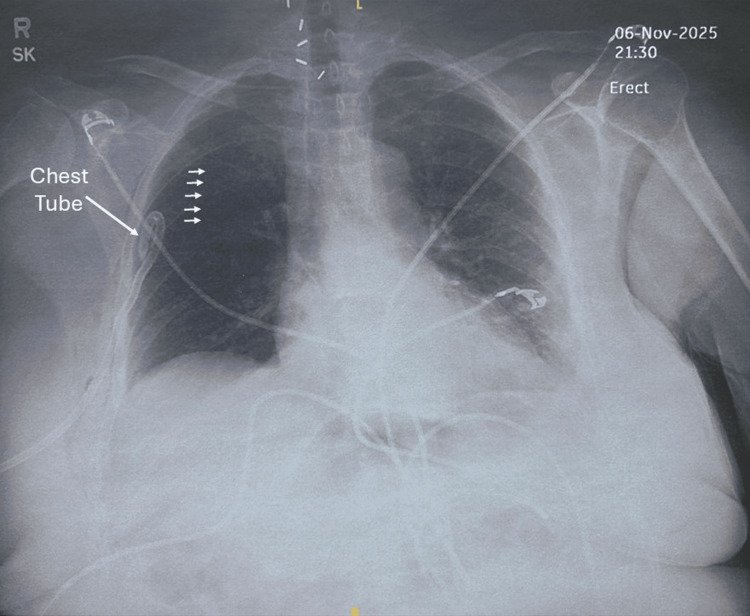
Chest radiograph demonstrating a large right-sided pneumothorax (small arrows) with pigtail chest tube placement
